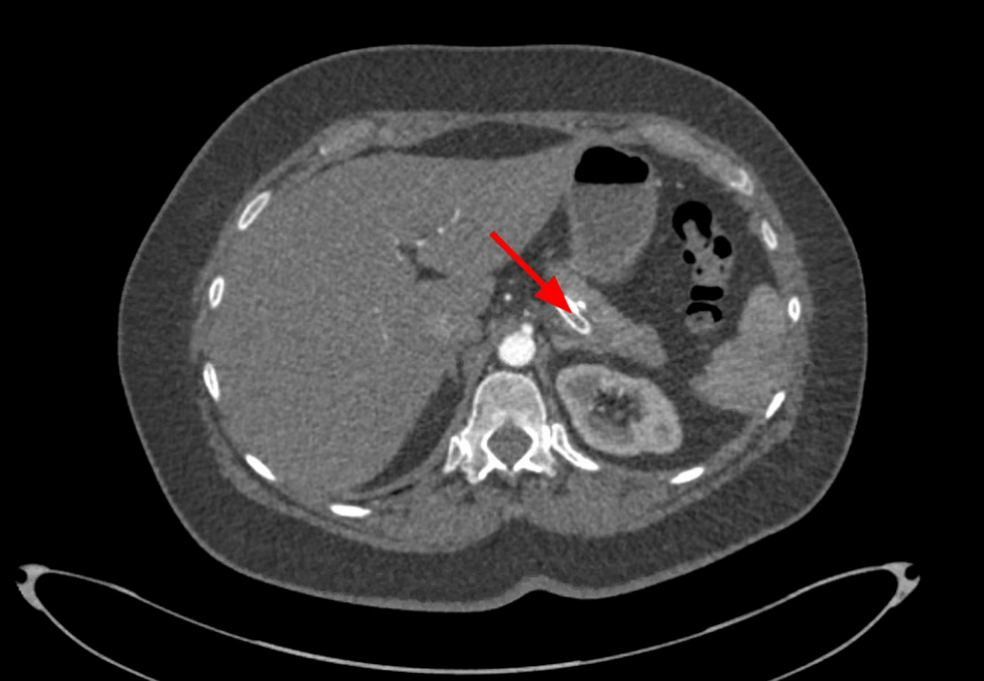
CTA abdomen and pelvis on POD#30 showing the splenic artery thrombosis (red arrow). CTA, computed tomography angiography; POD, post-operative day
